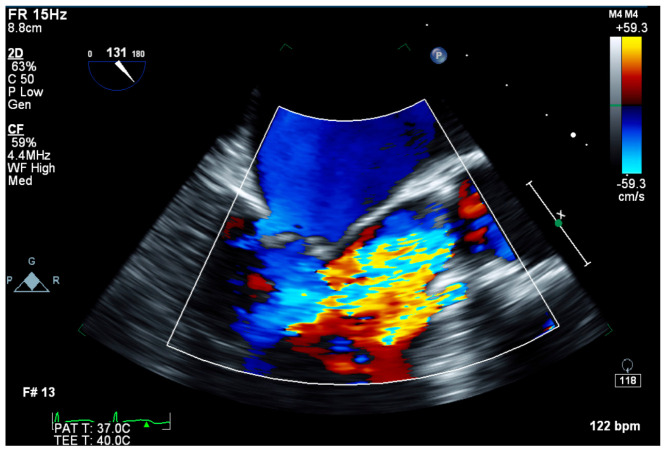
Transesophageal echocardiogram long axis view with color doppler demonstrating two turbulent jets, consistent with severe aortic insufficiency.
